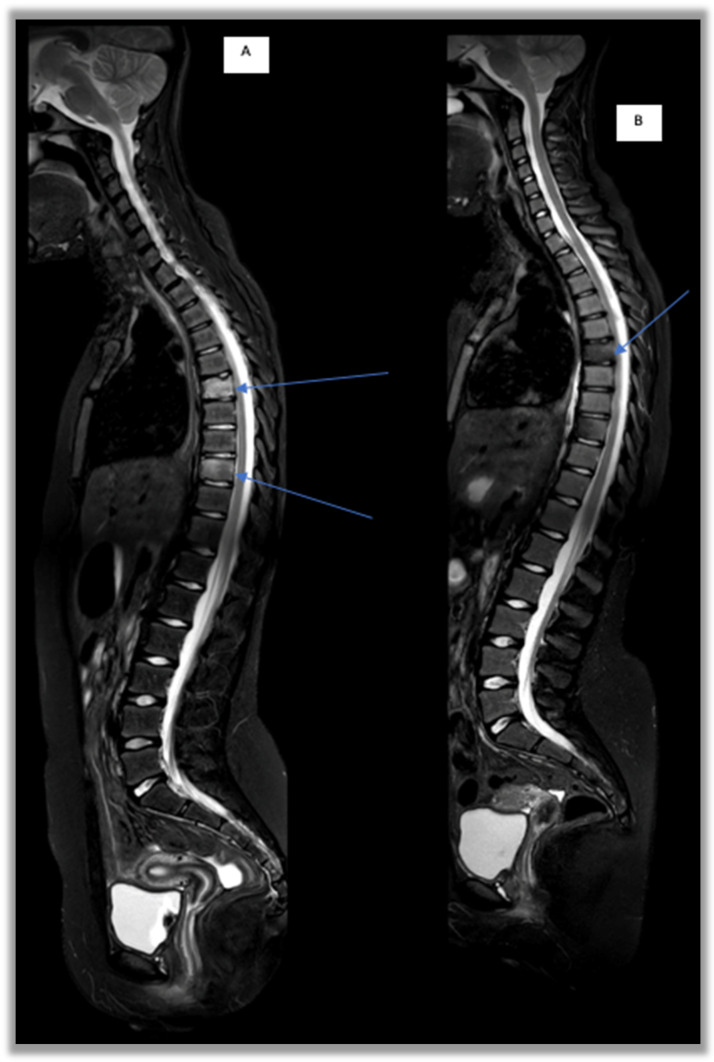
STIR sagittal sequences of the spine. D6 and D9: vertebral involvement in a CNO patient before and after pamidronate treatment. (A) D6 and D9 vertebral edema, MRI-WB T0 pre-pamidronate; (B) regression of bone marrow edema at T12 follow-up post-pamidronate.
